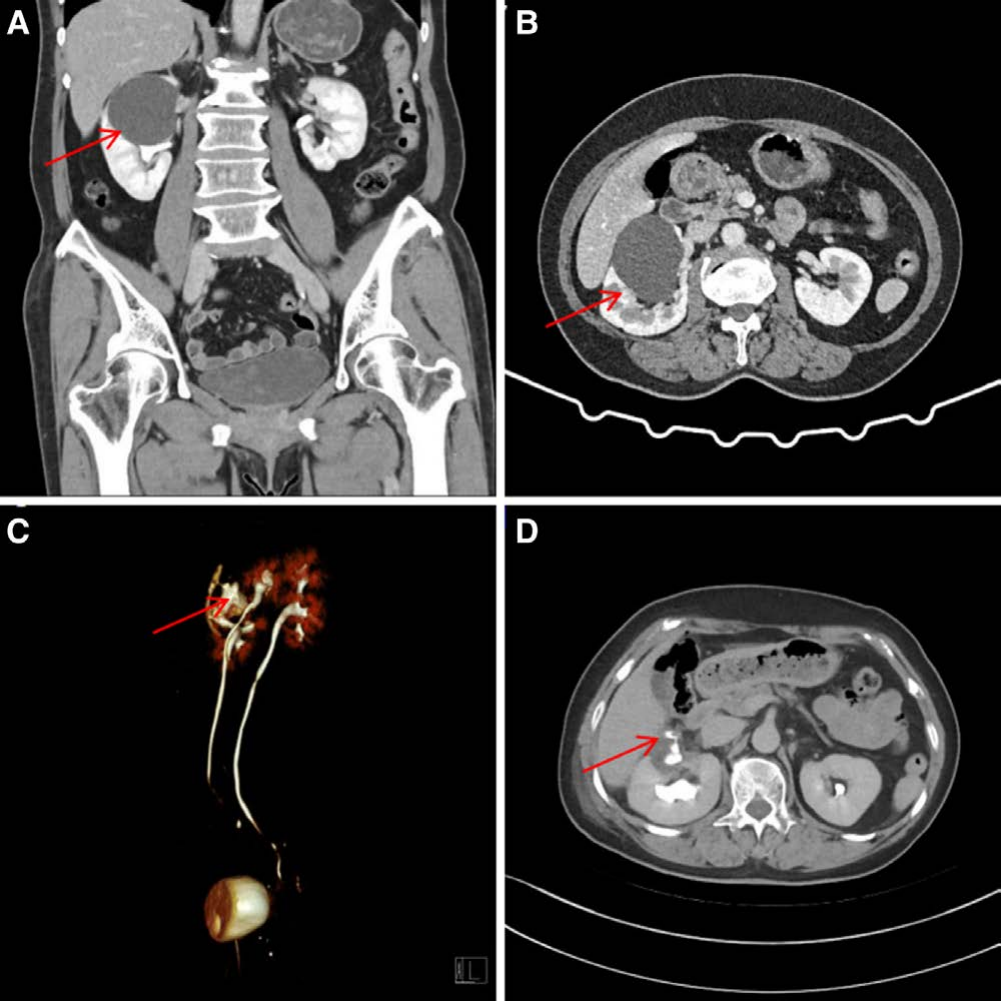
(A and B) Preoperative contrast-enhanced CT images demonstrate a hypodense cystic lesion in the mid-portion of the right kidney without contrast enhancement. (C and D) Postoperative CTU imaging findings. CT = computed tomography, CTU = CT urography.
